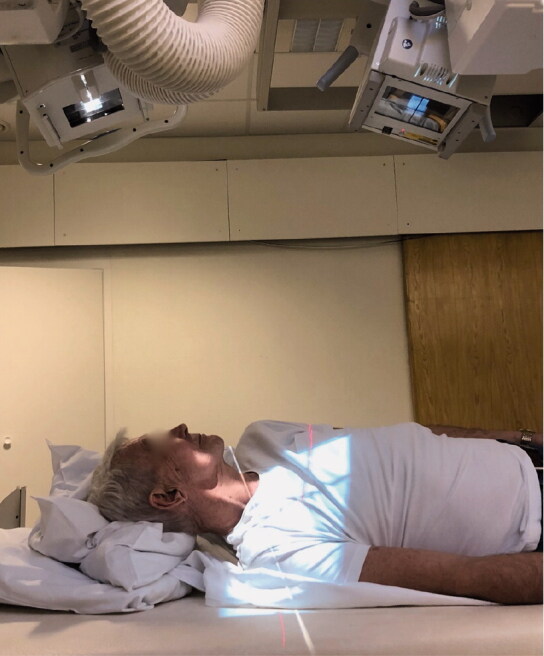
Patient positioning when performing shoulder RSA. The radiographic tubes were centered on the glenoid implant of the right shoulder and aligned along the length axis of the patient, resulting in a sagittal radiographic plane.
